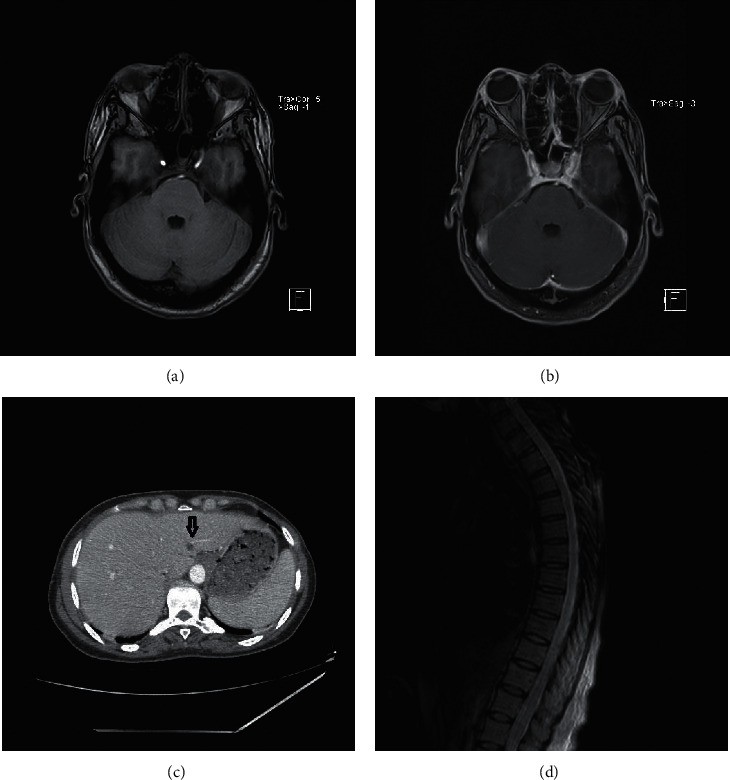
(a) MRI brain precontrast. (b) MRI brain postcontrast, showing dural enhancement. (c) CT chest, abdomen, and pelvis showing the hypoattenuating nodule in the liver (arrow). (d) MRI lower thoracic spine showing mild degenerative changes.
